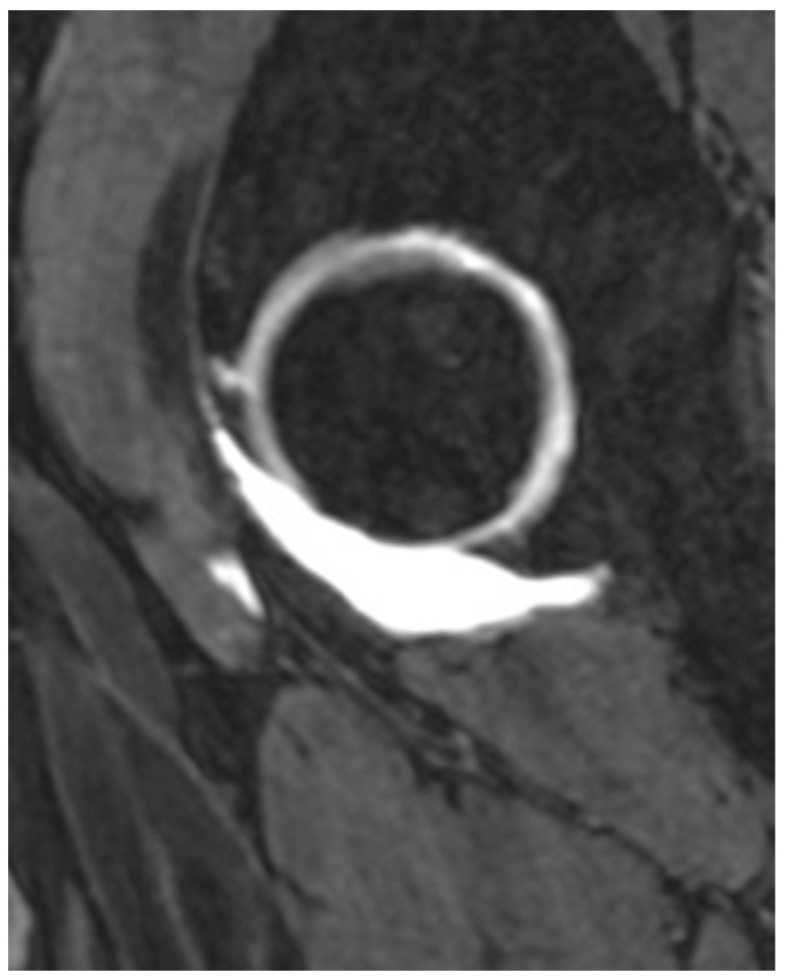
Arthro-MRI with labral tears.
